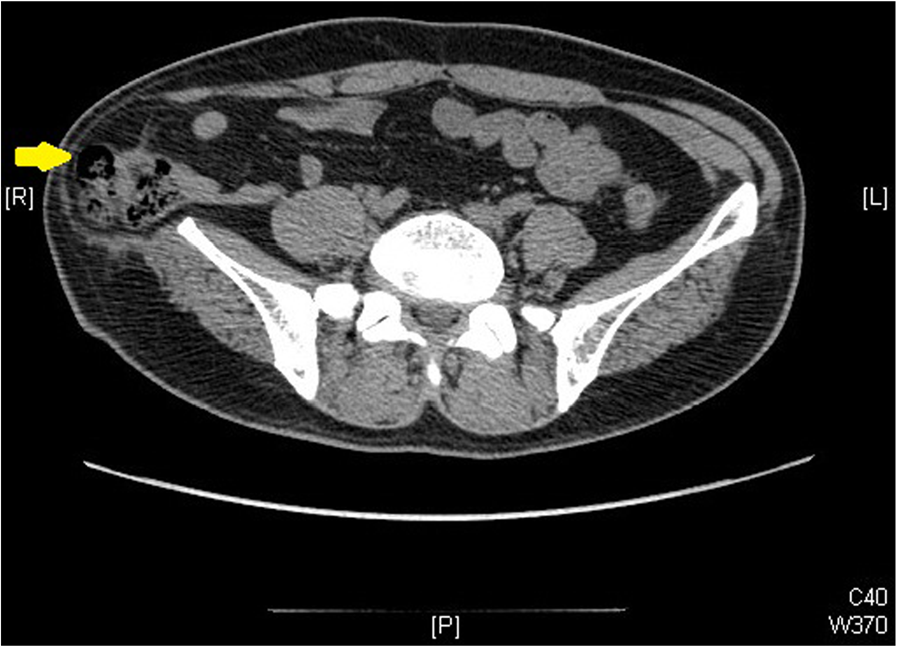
CT image (case 1). Herniation (*arrow*) can be observed through bone defect caused by DCIA flap harvesting. There are no anatomical barriers to avoid herniation
